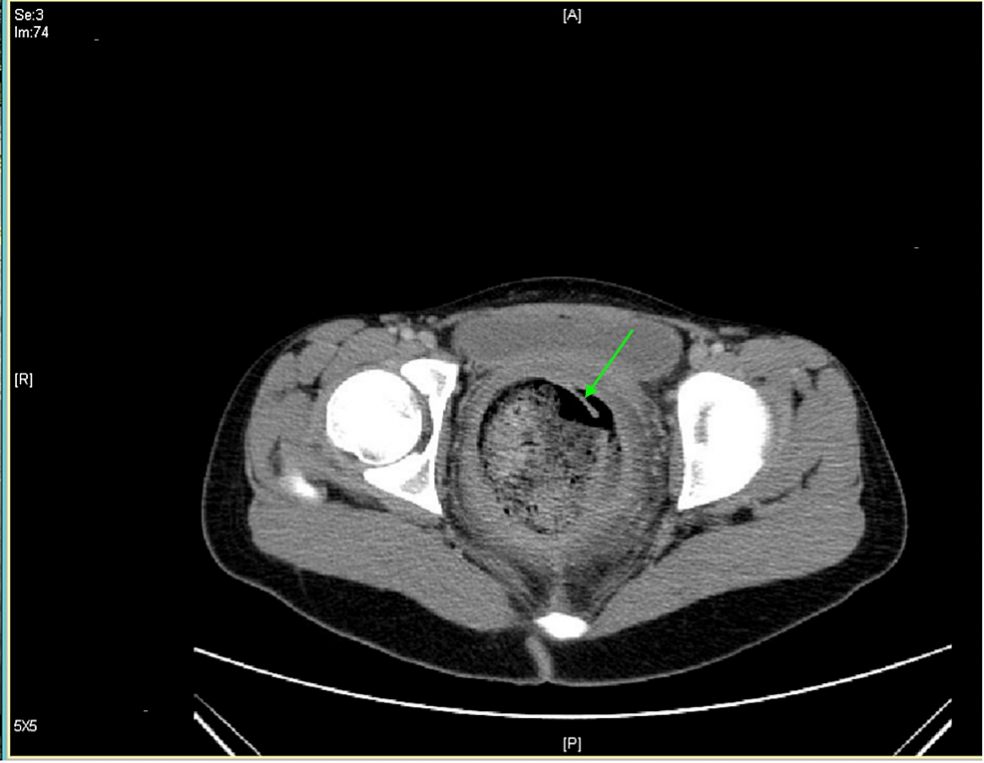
Sagittal computed tomography imaging showing rectal perforation (green arrow).
